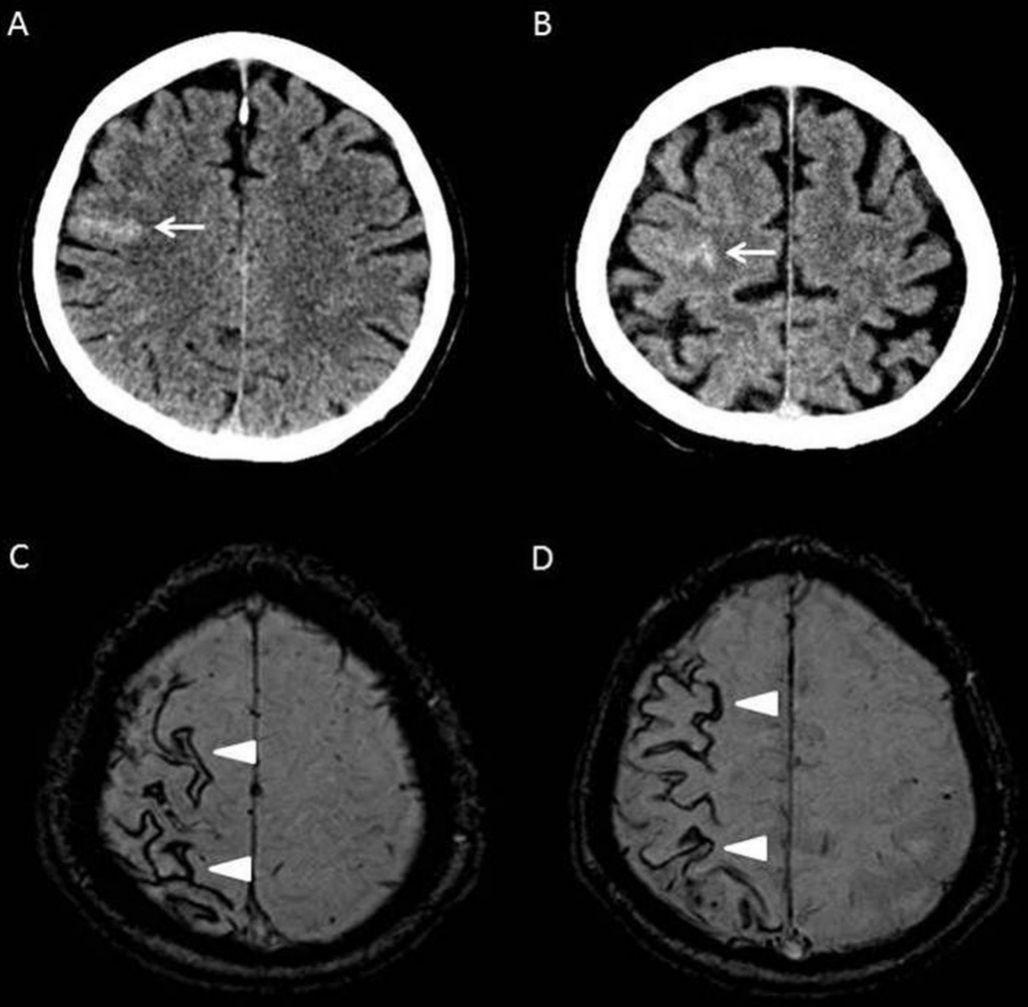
Imaging findings in CAA-associated TFNE. Images from a 76-year-old patient who presented with migratory left-sided sensory symptoms consistent with CAA-associated TFNE. His original CT (A) shows a hyperdense area in keeping with an acute cSAH (arrow). Three months later he had a similar episode; repeat CT (B) at this time demonstrated another acute cSAH nearby (arrow). Subsequent susceptibility weighted MRI (C and D) showed widespread disseminated cSS affecting the right hemisphere (arrowheads). CAA, cerebral amyloid angiopathy; cSAH, convexity subarachnoid haemorrhage; cSS, cortical superficial siderosis; TFNE, transient focal neurological episodes.
